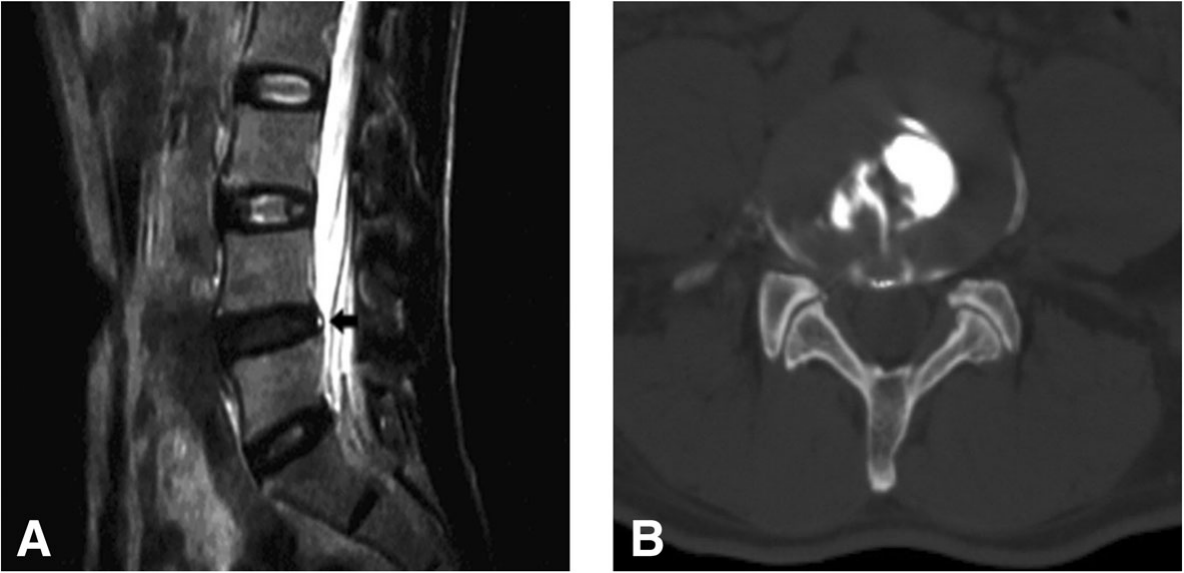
A 52-year-old man with low back pain for more than 1 year. a Sagittal T2-weighted MR image of the lumbar spine shows one HIZ at L4/5 (black arrow). b Axial post-discography CT image shows a grade 3 annular disruption at L4/5. Discography produced no pain
